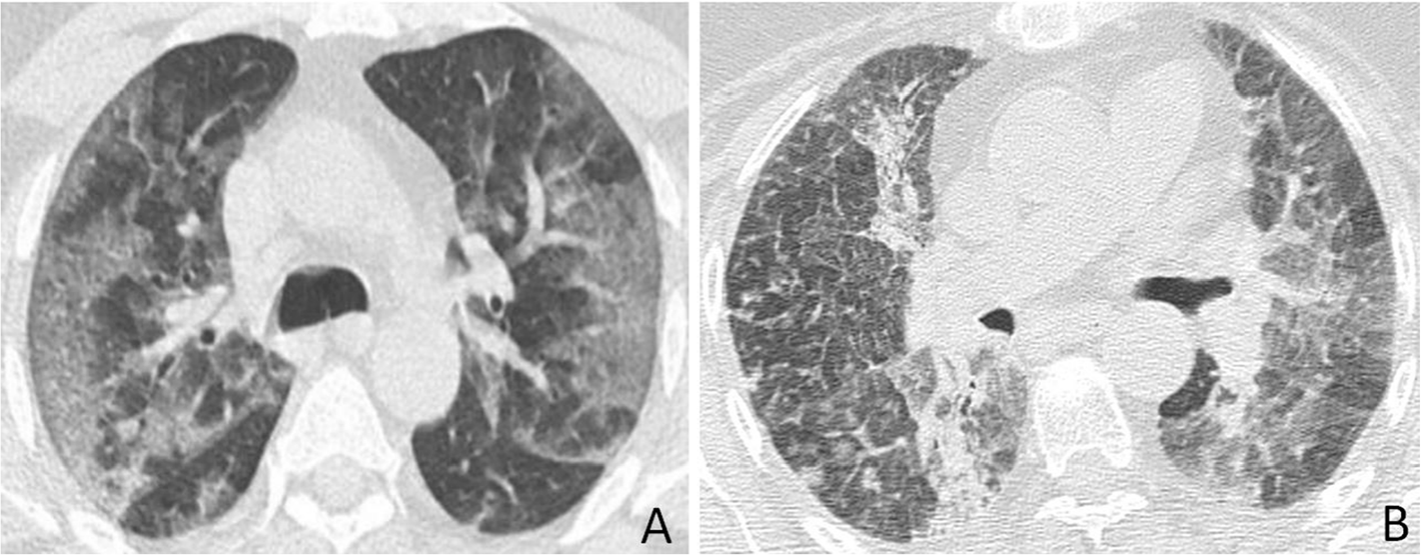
COVID-19 and drug-induced lung injury misdiagnosed as COVID-19. a Axial CT image obtained from a 62-year-old man with positive PCR test for COVID-19 shows multifocal peripheral GGO with superimposed interlobular septal thickening and visible intralobular lines (“crazy-paving”). b Bleomycin-induced lung injury in a 55-year-old woman with history of non-Hodgkin lymphoma; axial CT image shows multifocal areas of GGO, consolidations, and few pulmonary nodules in the right lung
